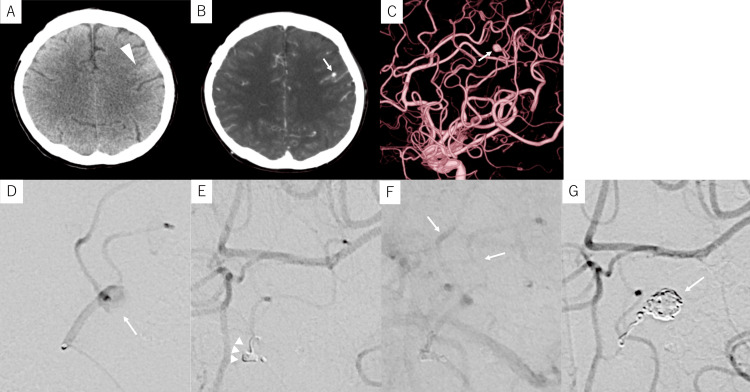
Preoperative and intraoperative imaging of Case 3 Case 3: A 59-year-old man with a small subarachnoid hemorrhage. (A) Computed tomography (CT) upon admission shows a small amount of subarachnoid hemorrhage in the left frontal sulcus (arrowhead). (B) Contrast-enhanced CT shows a small enhanced lesion (arrow) within the hematoma. (C) Digital subtraction angiography (DSA) of the left internal carotid artery (lateral view) shows a 5.0-mm fusiform aneurysm (arrow) on the peripheral branch of the left middle cerebral artery. (D) Selective angiography of the parent artery (working-angle view) shows a fusiform aneurysm (arrow). (E, F) DSA of the left internal carotid artery while performing an occlusion test of the parent artery with a coil (arrowheads): (E) Occlusion of the parent artery in the arterial phase; (F) Delayed filling downstream (arrows) in the venous phase. (G) Post-embolization DSA of the left internal carotid artery shows complete occlusion of the aneurysm (arrow).
